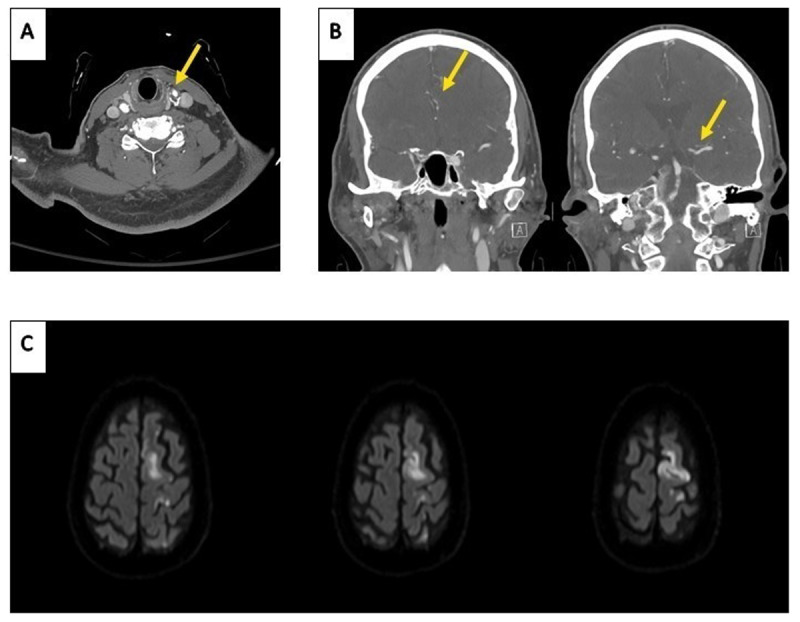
Computed tomography angiography (CTA) of the head and neck and brain magnetic resonance imaging (MRI). (A) Axial CTA of the neck showing soft plaque with up to 50% stenosis at the left carotid bulb/proximal left internal carotid artery, (B) Coronal CTA of the head showing A2 segment of the left anterior cerebral artery (ACA) stenosis and left medial cerebral artery (MCA) irregularities, (C) Diffusion-weighted imaging (DWI) showing acute left anterior cerebral artery (ACA) infarction involving the frontoparietal regions, including the precentral gyrus.
